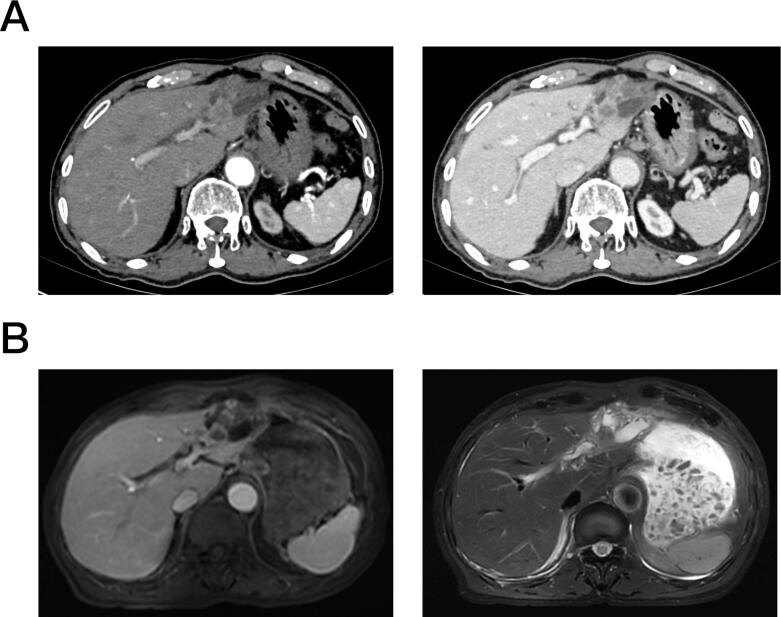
Preoperative abdominal contrast-enhanced CT (A, left: arterial phase, right: venous phase) and MRI (B, left: T1WI, right: T2WI) (April 2024) showing a 1.2 cm × 1.2 cm mass in the left lateral segment of the liver with heterogeneous enhancement and intrahepatic bile duct dilation.
